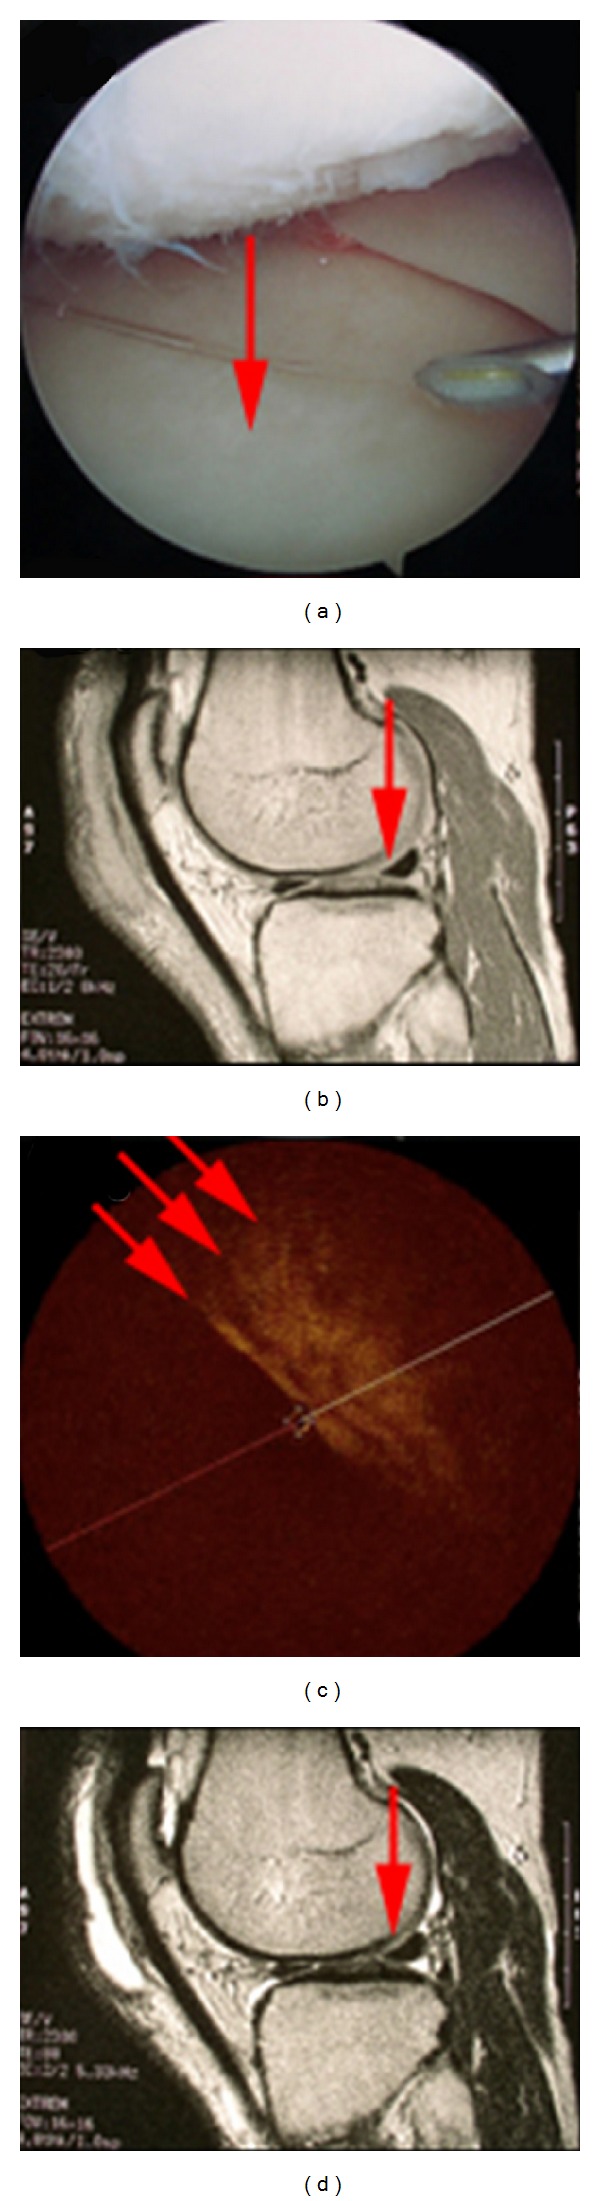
(a) Arthroscopic image of normal medial tibial cartilage. The red arrow represents a section of smooth normal looking cartilage. (b) and (d) MR image of the joint using T1 and T2 (top to bottom) relaxation states. The sagittal cross-section shows a uniformly homogeneous articular layer at the indicated point. (c) OCT image of area represented by the arthroscopic image. The OCT image shows a uniform banding pattern, indicative of healthy cartilage. Image courtesy of Zheng et al. [9].
